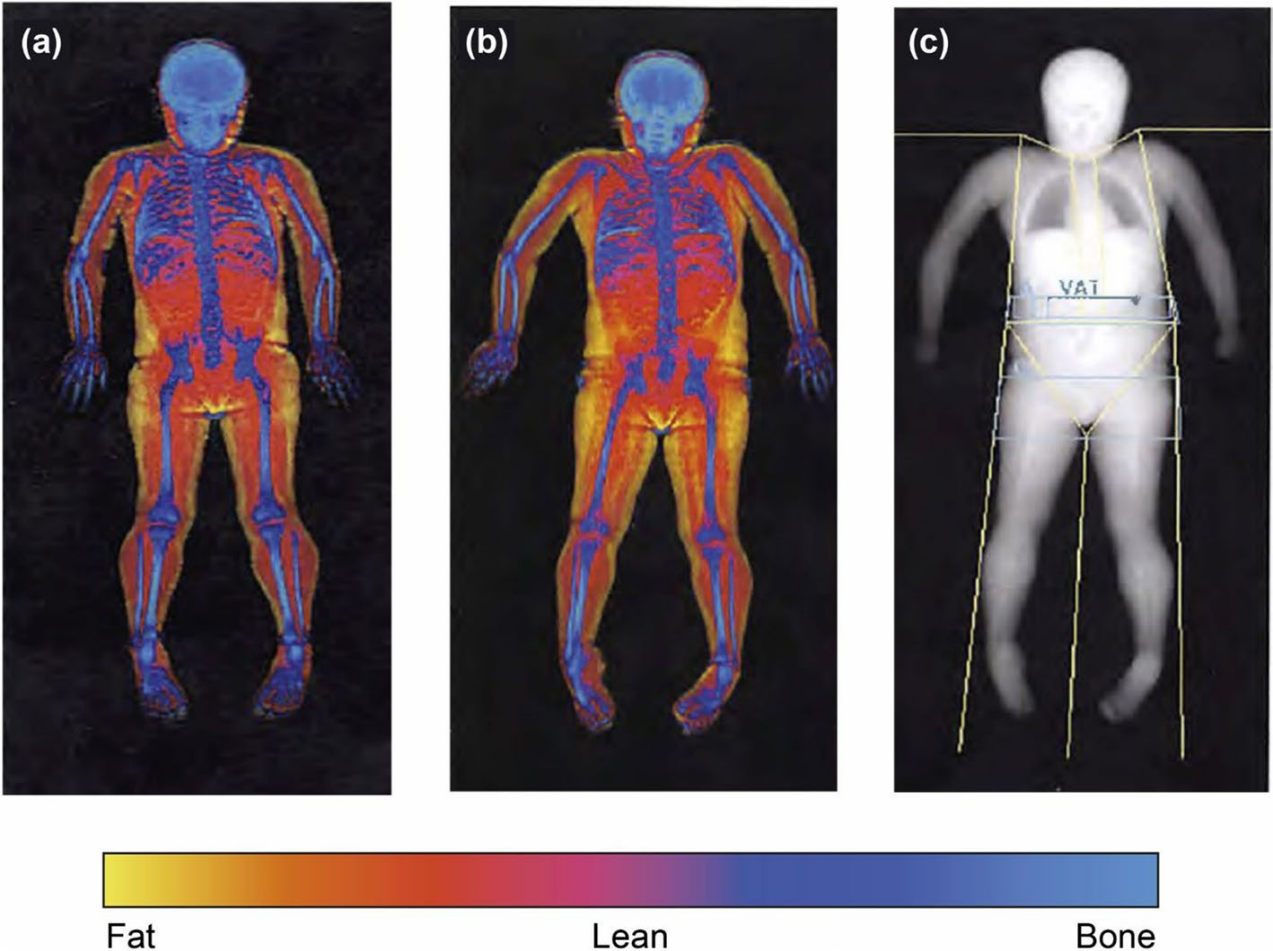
Representative DXA scan. A visual representation of fat mass, lean body mass and bone mass is shown for a participant (a) before treatment and (b) at the Week 49 visit. Cutlines are placed on the DXA scan to separate regions of interest for analysis (c). Primary regions for analysis included arms (left and right combined), legs (left and right combined), appendicular skeleton (arms and leg as a single region of interest) and total body excluding the head. Cutlines were placed by a trained analyst and reviewed by a radiologist prior to finalizing read results. DXA, Dual-energy X-ray absorptiometry.
